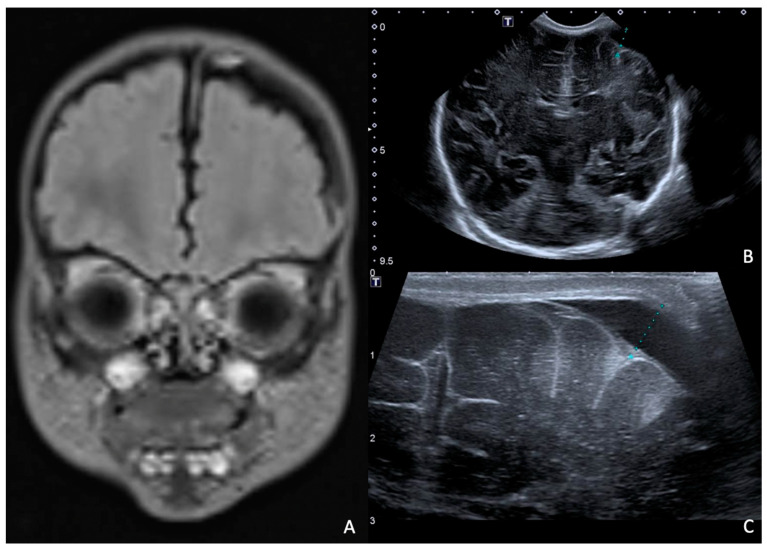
(A), Brain MRI in FLAIR sequence with evidence of left parietal subdural collection and multiple diffuse supra- and infratentorial subcentimetric collections. (B,C) HUS: left parietal subdural collection, with maximum thickness of 7 mm.
